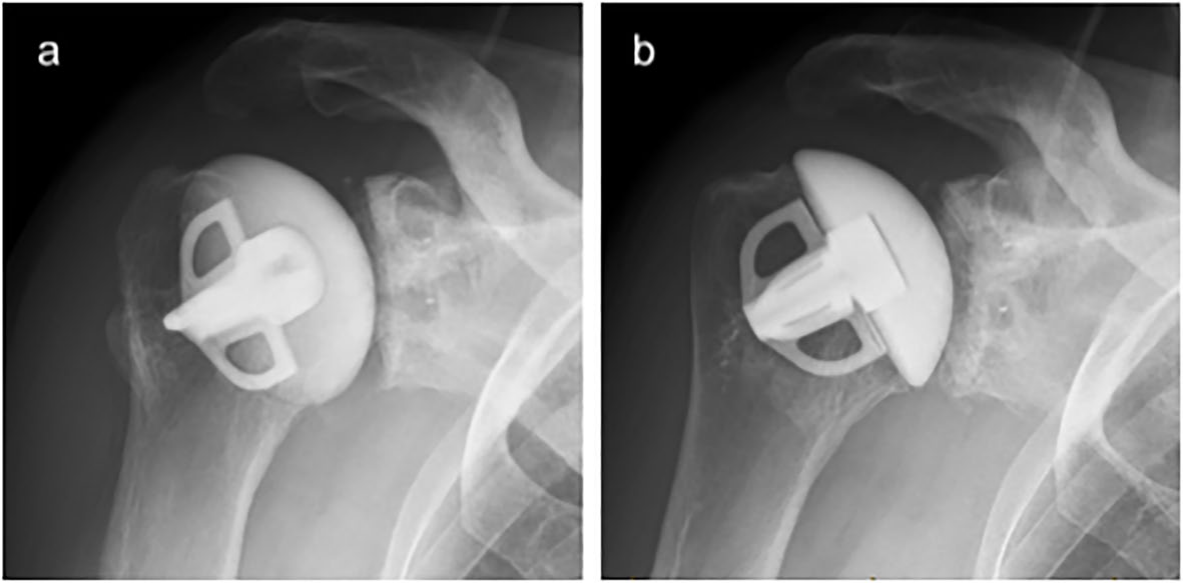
X-ray images, part a and b (true ap with the arm in 20° internal and external rotation, respectively), demonstrate the importance of multiple angles in order to properly assess the glenoid and the humeral component of the prosthesis. Glenoid component radiolucency is graded by the Lazarus score [17].
